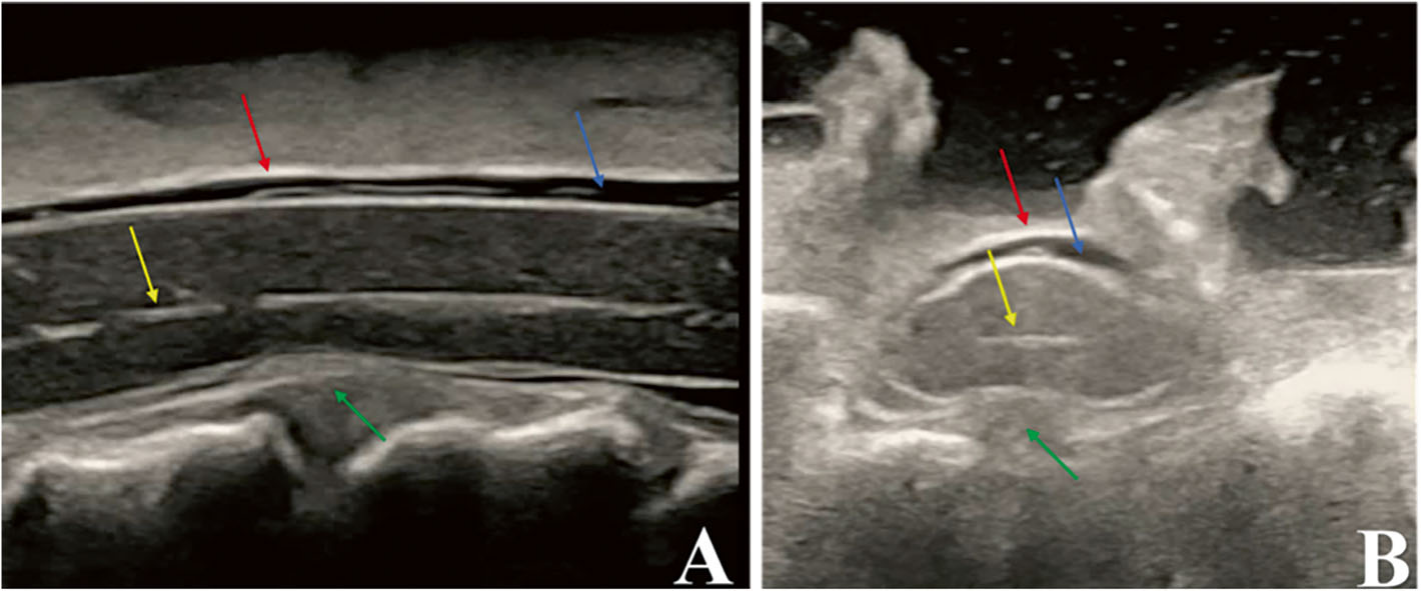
The anatomy of the spinal cord observed by intraoperative ultrasound. a) Midsagittal image, (b) Axial image. Red arrows indicate the spinal dura mater, yellow arrows indicate the central canal, blue arrows indicate the subarachnoid space and green arrows indicate the cervical disc
